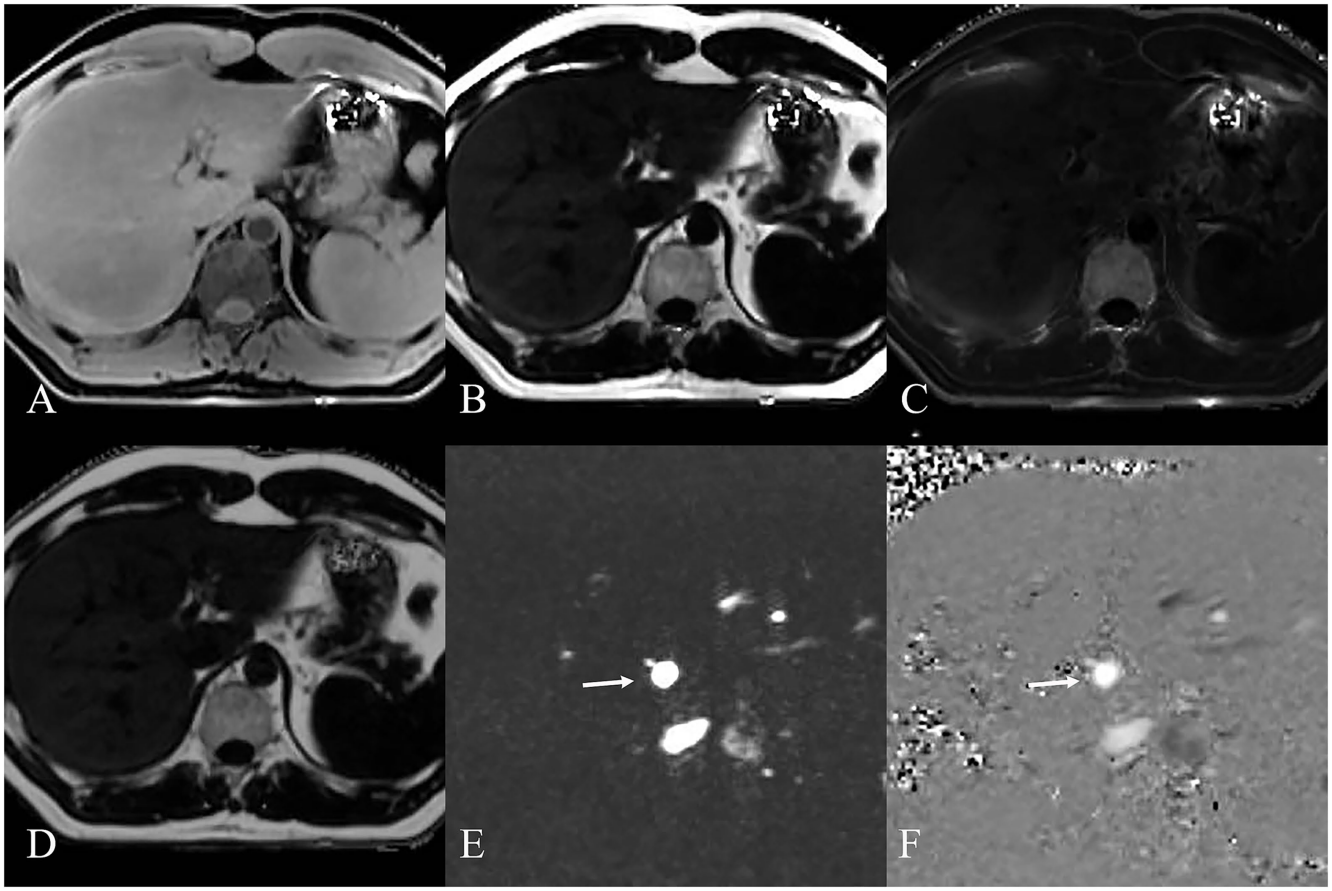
MRI images displaying various sequences used for MRI PDFF (A–D) and MRI 4D Flow (E,F) measurement of NAFLD. (A) Water image shows the water content in the liver. (B) Fat image shows the fat distribution in the liver. (C) R2* map shows the liver relaxation rate. (D) Fat fraction map shows the liver fat content quantifying. MRI 4D Flow magnitude image (E) and phase difference image (F) show the hepatic hemodynamics with flow visualization in the portal vein (white arrow).
